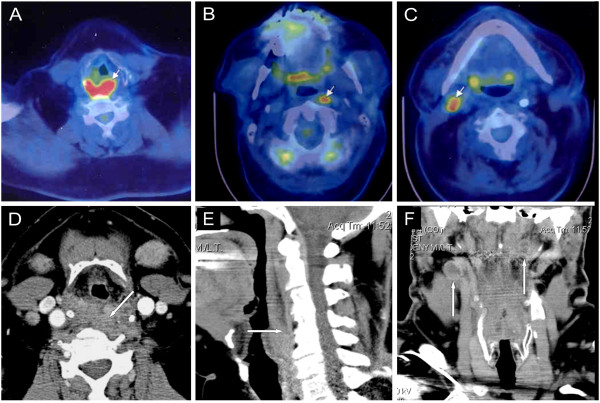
Hypopharyngeal cancer with bilateral metastatic lymph nodes - was performed to detect the site of an unknown primary tumour. On axial plane PET/CT images: A, B, C, the examination revealed cancer on the dorsal pharyngeal wall (arrow), and suspected the presence of metastatic lymph nodes in the deep cervical lymphatic regions bilaterally (arrows). Multiplanar Contrast-enhanced-CT images: D, E, F scans in axial, sagittal and coronal planes also demonstrate a mass in the dorsal wall of the hypopharynx, that affects the integrity of the fat layer in the prevertebral space, clearly detectable on sagittal plane image (arrow). Coronal CT scan shows metastatic lymph nodes bilateraly (arrows) with colliquation, however they are less detectable as by PET/CT. Estimated stage by CT: T4b N2c.
